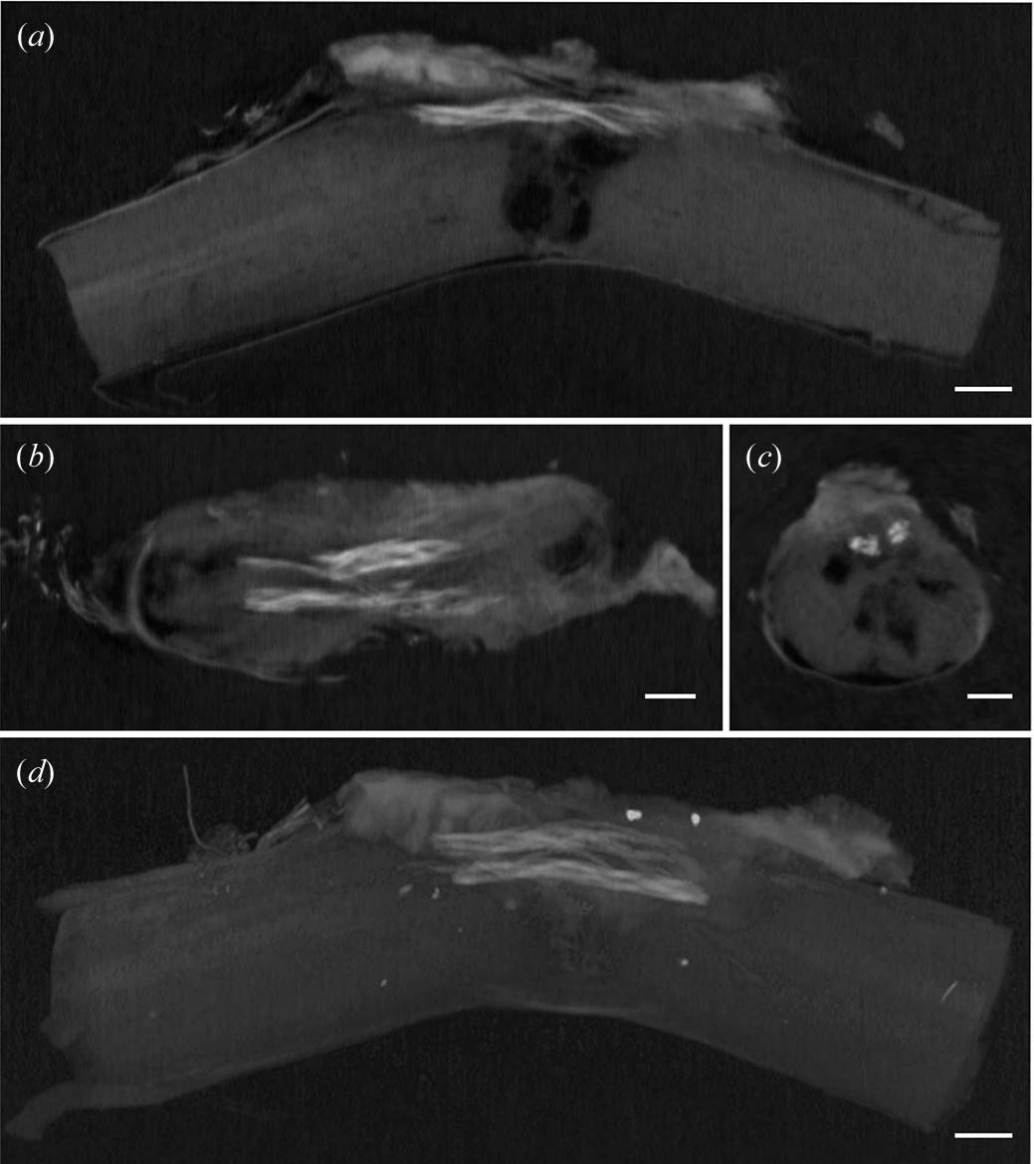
Microstructure of braided scaffolds in the injured spinal cord was visible on X-ray phase-contrast CT with a synchrotron radiation source. (*a*–*c*) X-ray phase-contrast CT images of the injured spinal cord 28 days after scaffold implantation in sagittal (*a*), horizontal (*b*) and coronal slices (*c*). Note the preserved tubular structure of the braided scaffold. (*d*) A three-dimensional image rendering of the injured spinal cord 28 days after scaffold implantation. Scale bar = 500 µm.
